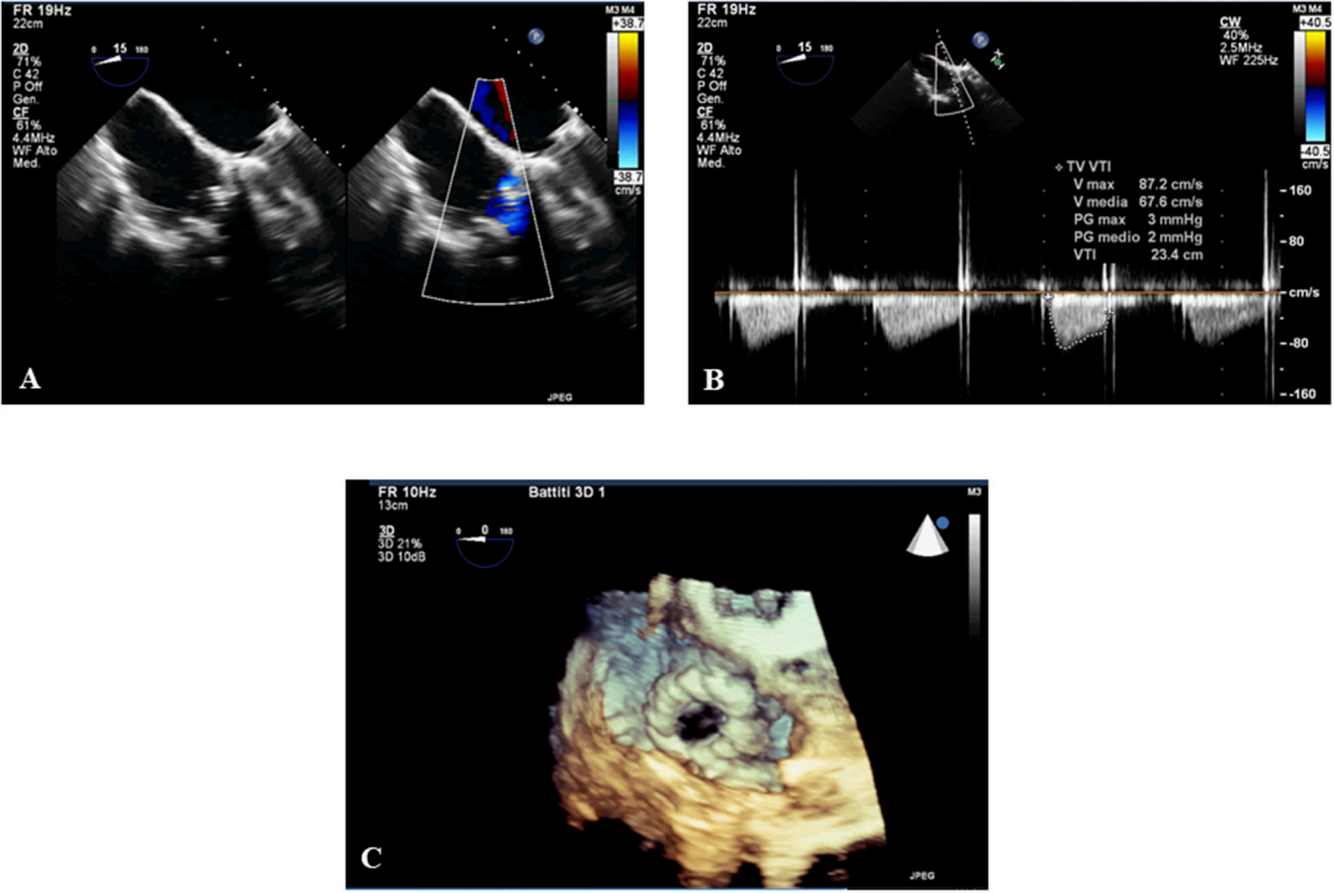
The 3D transesophageal echocardiography (TEE) post procedure shows the normal function of the tricuspid valve. The 3DTEE post procedure shows the absence of regurgitation (A), stenosis (B), and the 3D reconstruction (C) of the tricuspid prosthesis after the valve in valve intervention.
